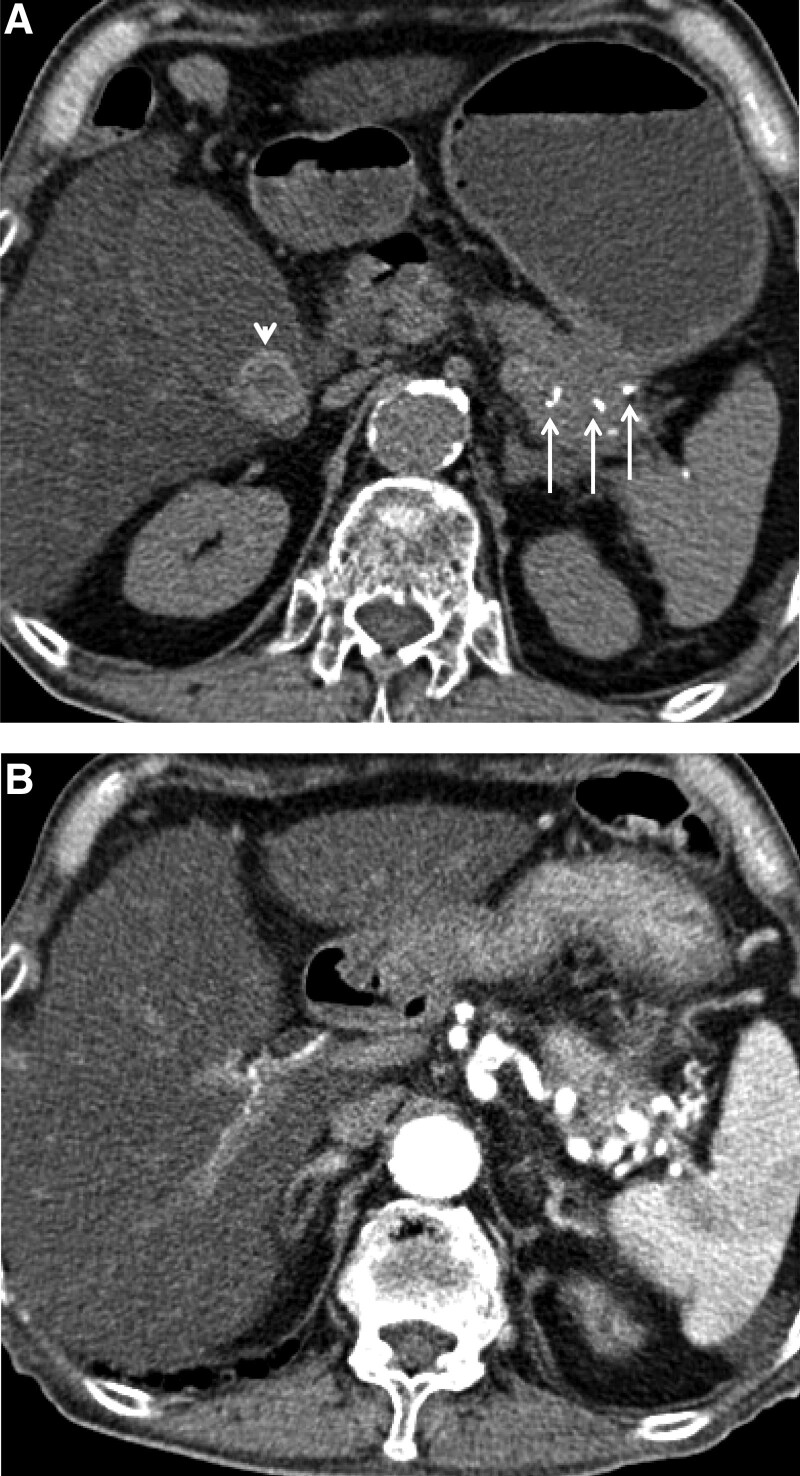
Contrast-enhanced computed tomography (CT) scans. A: Pre-contrast phase CT scan showing a gallbladder stone (arrowhead) and pancreatic calcifications (arrow); B: Arterial phase CT scan showing the tortuosity of the splenic artery. CT = computed tomography.
